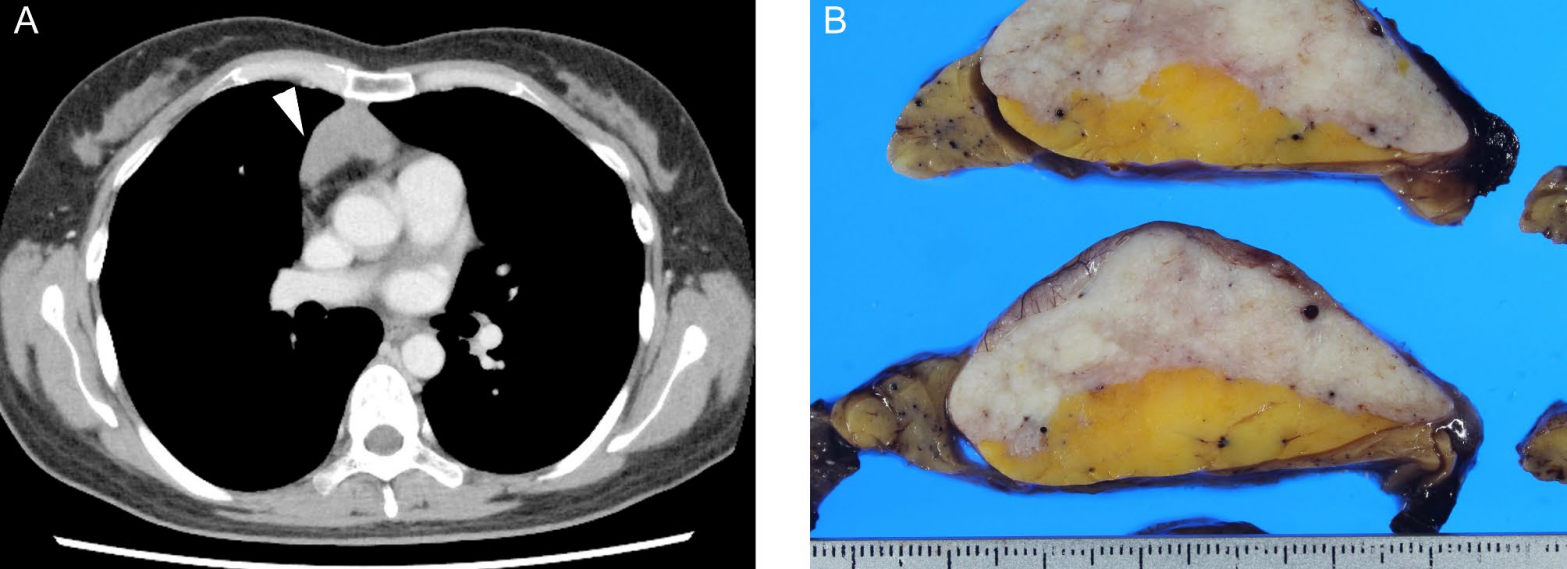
Clinical images: (A) Chest computed tomography revealed a non-contrast-enhanced mass lesion in the anterior mediastinum. (White arrowhead) Macroscopic images: (B) The cut surface of the material in the anterior mediastinal tumor showed a yellowish lipomatous component and a uniform whitish fibrous component
